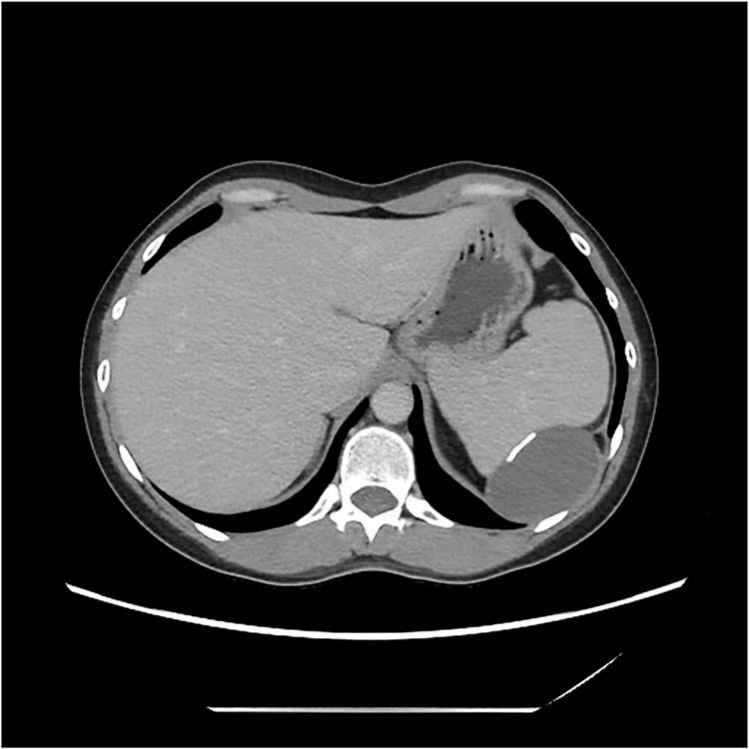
CT image demonstrating cyst adjacent to spleen and diaphgram with calcified walls.
